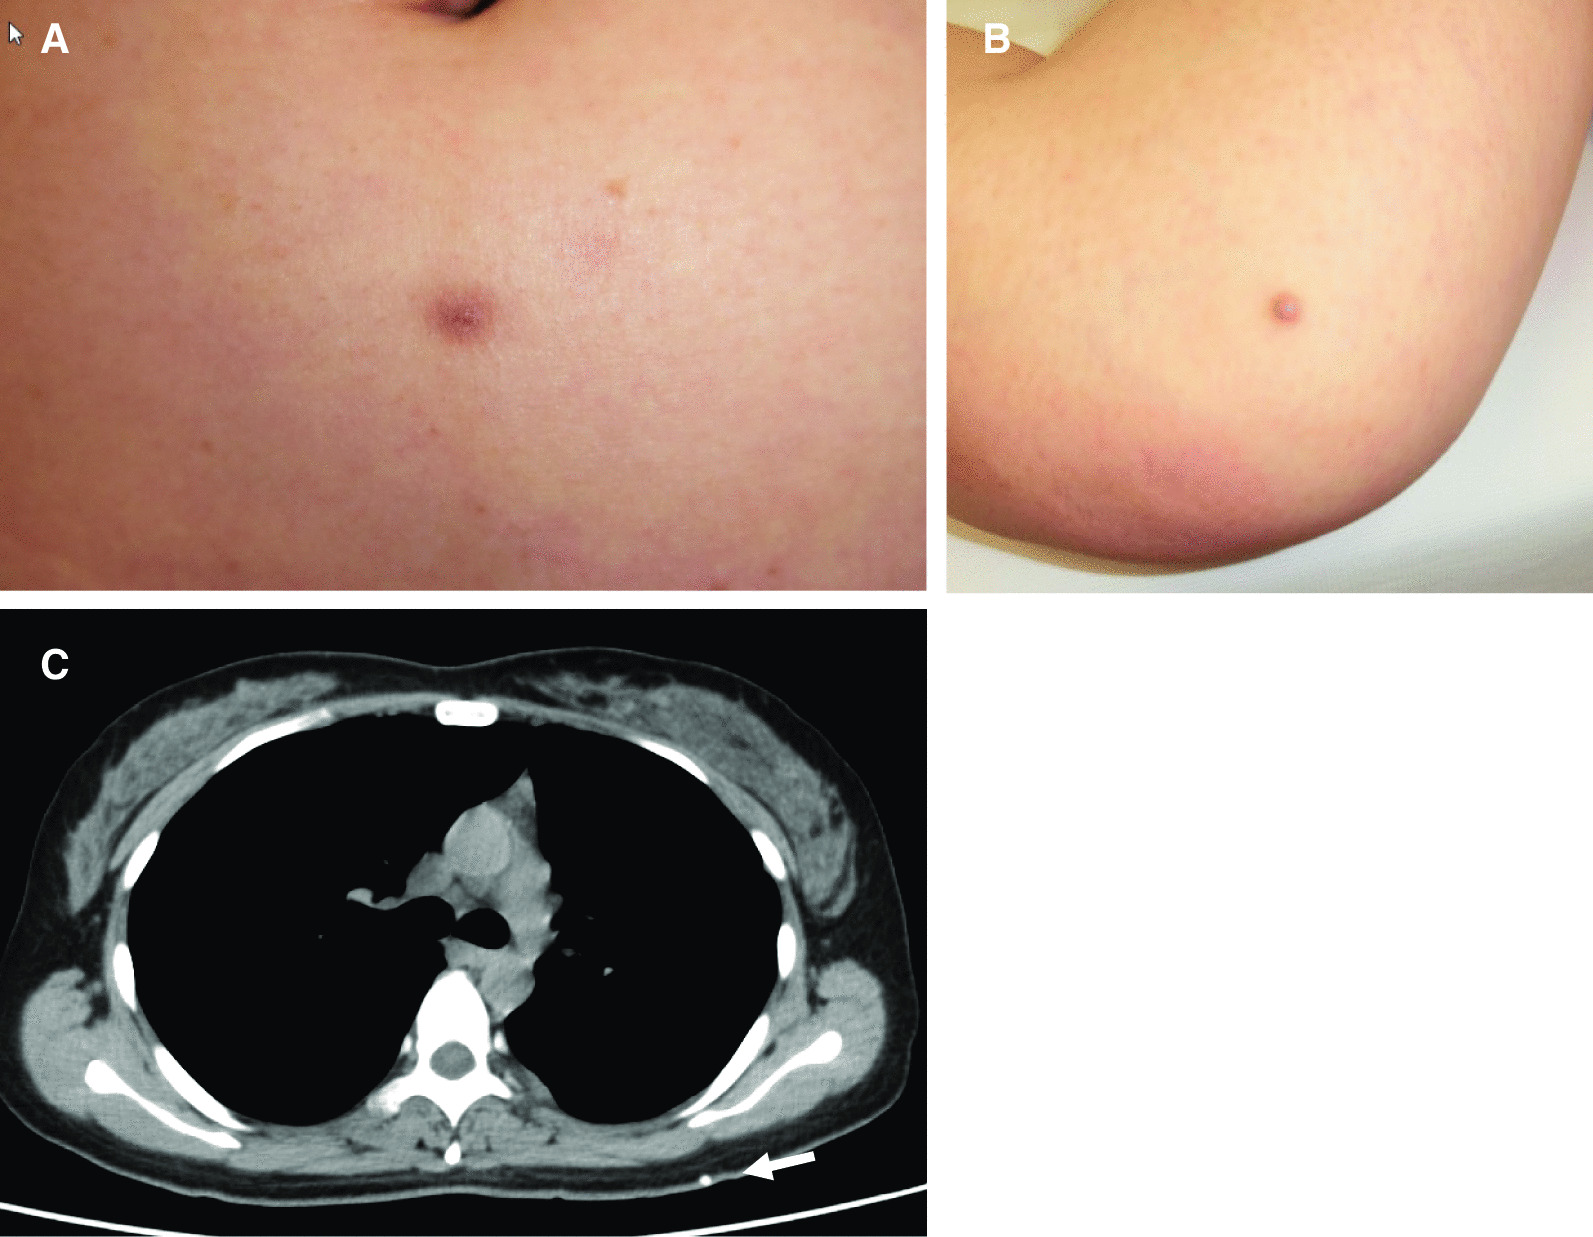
A, B On physical examination, more than 10 subcutaneous lesions were palpable on the chest, back, lower abdomen, and right knee. C Chest CT scan showing a calcified lesion high on the back (arrow)
